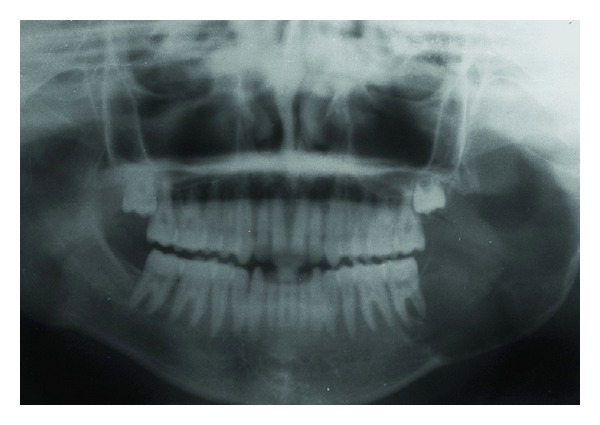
Panoramic radiograph showing the tumor at the left ramus of the mandible.
